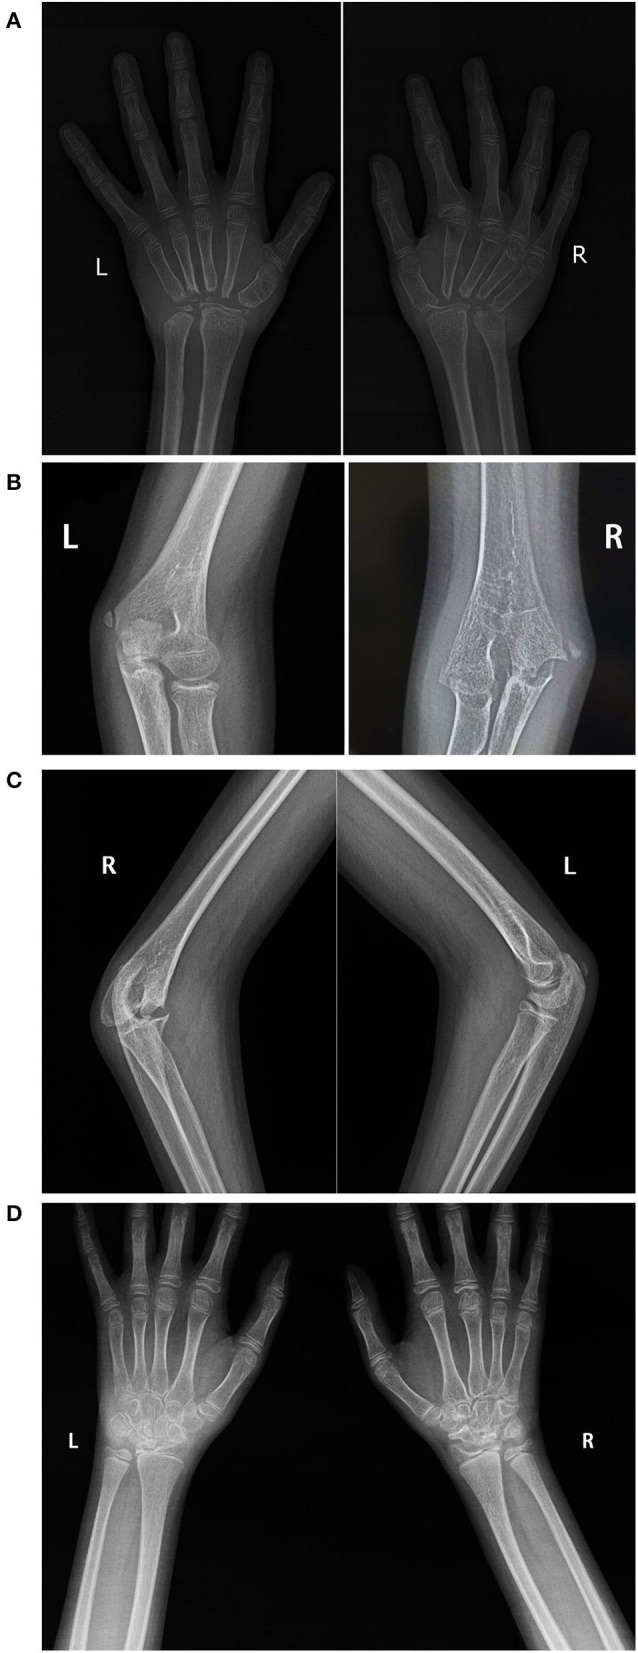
Radiological findings. X-ray of the hands of patient 1 (A), taken at 12 years old, showing severe erosion of the carpal bones and the proximal metacarpal bones. X-ray of the elbows of patient 1 (B,C), showing deformation of the distal right humerus and proximal ulnar and radius; destruction of the ossification center, narrowing of the space in the right elbow joint, and swelling of the soft tissue around the right elbow joint. X-ray of the wrist of patient 2 (D) showing abnormal bilateral carpal bone morphology, especially the proximal row of carpal bones.
